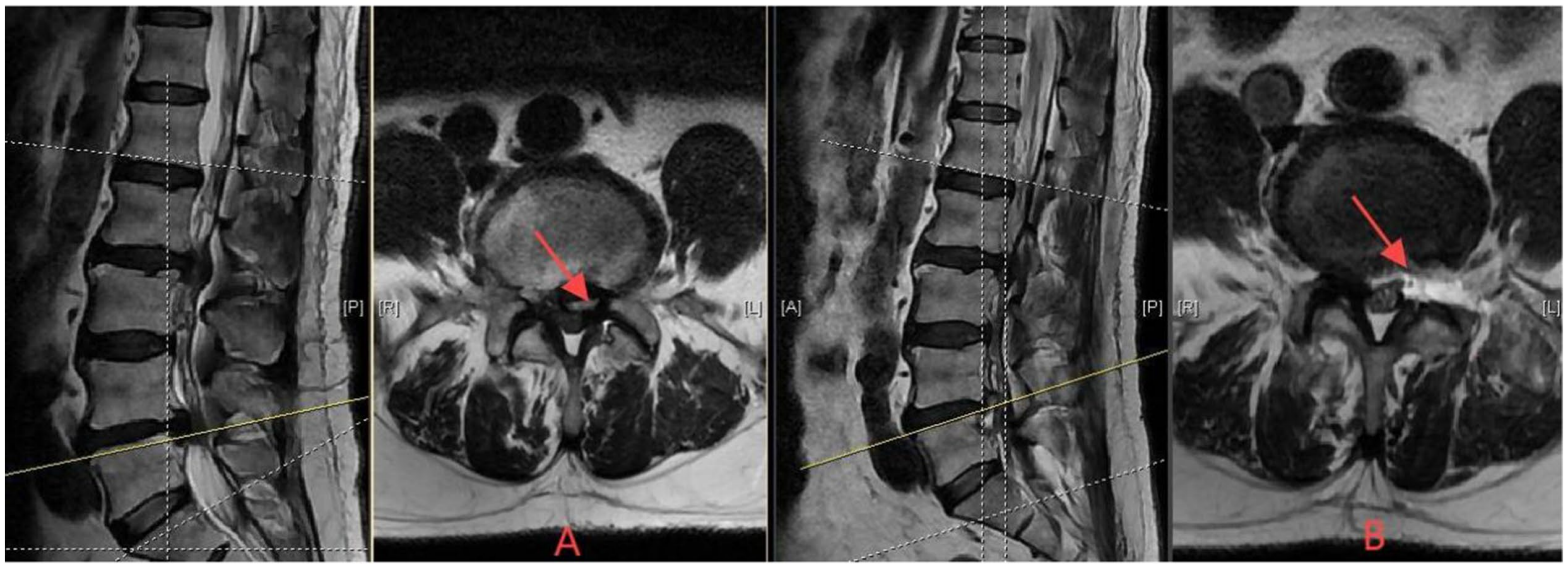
Axial T2-weighted MRI demonstrating aggressive discectomy approach for L4/5 disc herniation. (A) Preoperative image showing posterolateral disc herniation with nerve root compression (red arrow). (B) One-week postoperative image following comprehensive removal of herniated disc and extensive nucleus pulposus extraction with systematic disc space exploration. Note extensive tissue removal and enlarged disc space (red arrow indicates decompressed area), demonstrating the comprehensive removal surgical strategy with neurological improvement.
